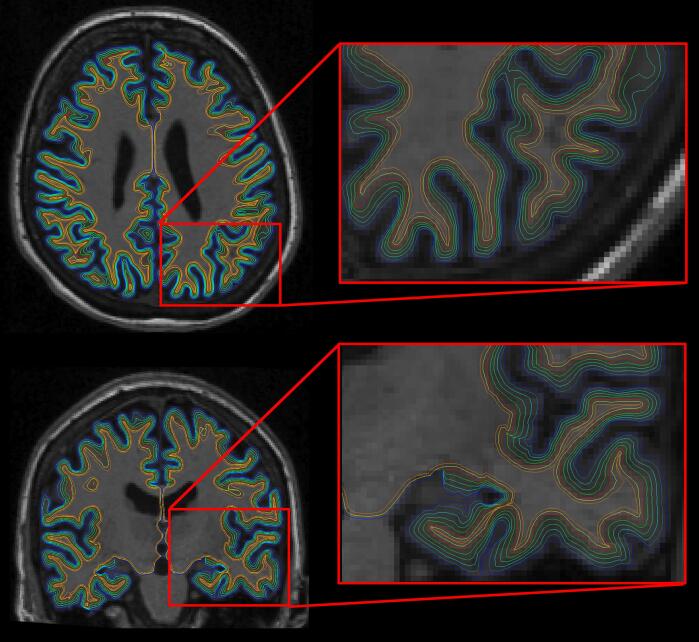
Microstructural feature map generation using a structural T1-weighted image. We expanded the intensity/contrast metrics to include tissue sampling from multiple points through the thickness of the cortical ribbon and subjacent white matter to obtain an array of intensity-linked features. Gray matter intensities were measured at depths of 20%, 40%, 60%, and 80% (surfaces between the blue and red) through the thickness of the cortical ribbon, starting from the gray/white border (red surfaces) towards the gray/CSF border (blue surfaces). White matter intensities were measured 0.5 mm and 1 mm subjacent to the gray/white border (surfaces underneath the red). (For interpretation of the references to color in this figure legend, the reader is referred to the web version of this article.)
